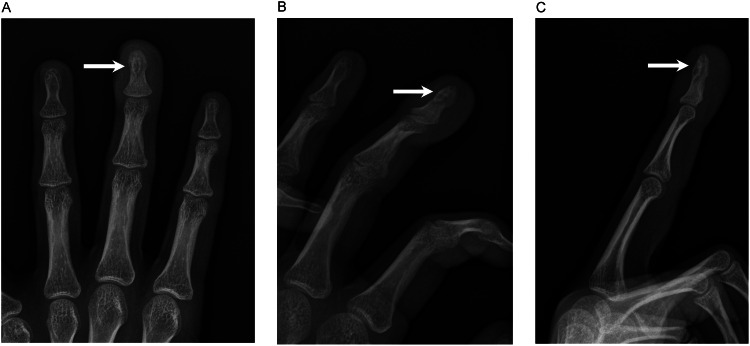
(A, B, C) Frontal, oblique, and lateral radiographs of the left middle finger show a lucent lesion with sclerotic margins in the left middle finger distal phalanx tuft.
